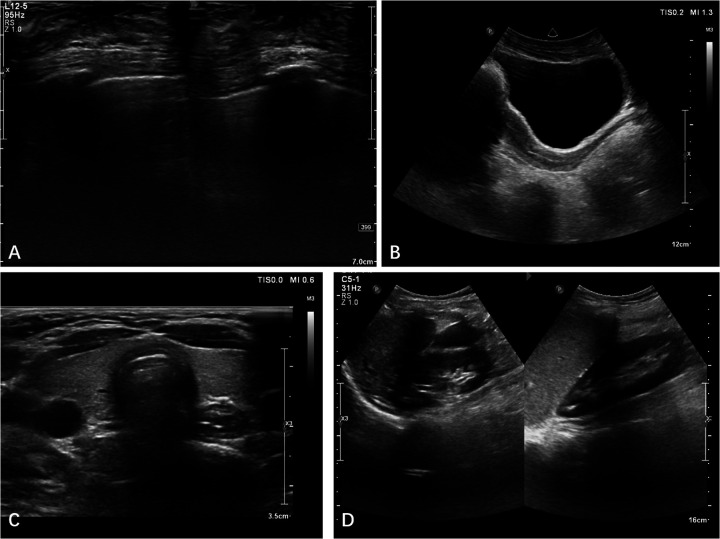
Ultrasound revealed bilateral breast enlargement (A) and enlarged uterus (B), while thyroid (C) and adrenal glands (D) showed no abnormalities.
